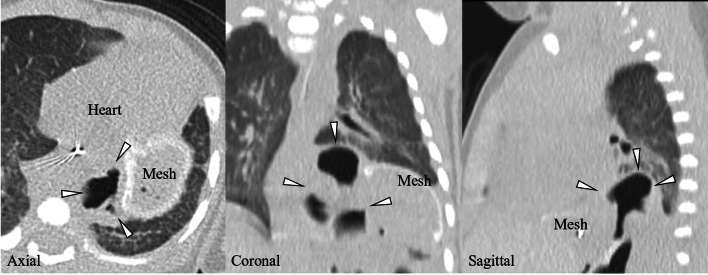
Computed tomography in case 1. Computed tomography shows intestine or colon is prolapsed into the thoracic cavity at the medial side of artificial mesh (arrowhead)
